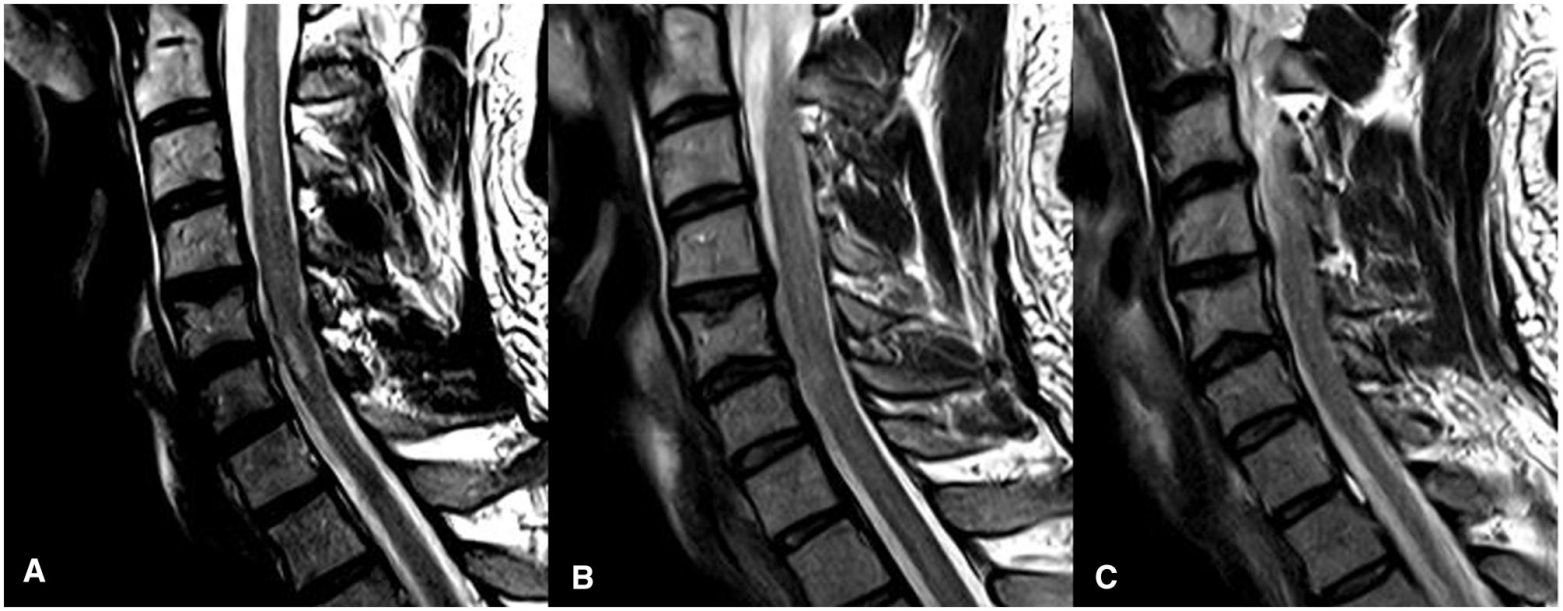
T2-weighted MRI of cervical spinal cord imaged at (A) right hemi-cord, (B) midline, and (C) left cervical hemi-cord demonstrating isolated FLAIR hyperintensity in the right cervical hemi-cord.
